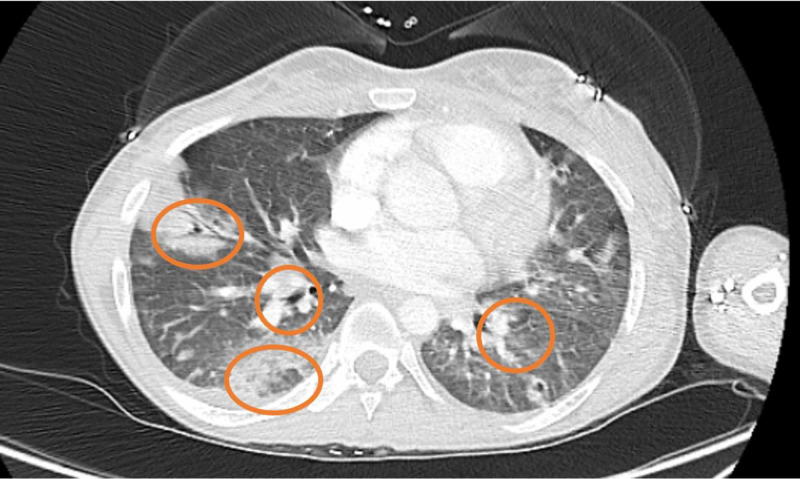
CT scan of chest with IV contrast. Axial view of chest CT (lung window) revealing multiple bilateral cavitary lesions due to septic emboli likely from tricuspid valve vegetations.
